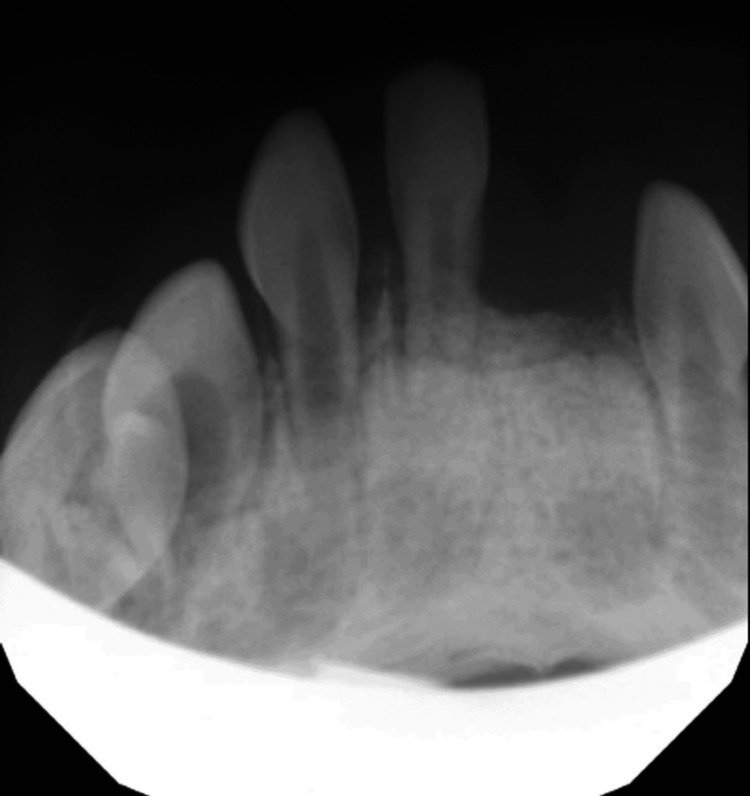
A mandibular occlusal radiograph was taken on the day of surgery
